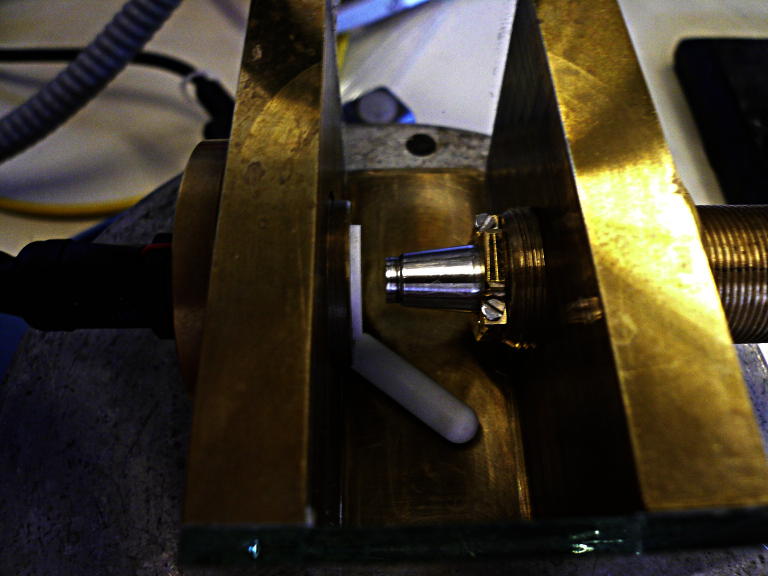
Experimental chamber setup made of brass; on the left hand side the Macor part of the ultrasonic transducer is visible. Facing it, the ATR probe is mounted onto the right wall of the chamber. By turning the fitting, the distance between the probe and the transducer can be adjusted. The white object at the bottom of the chamber is the magnetic stirrer.
